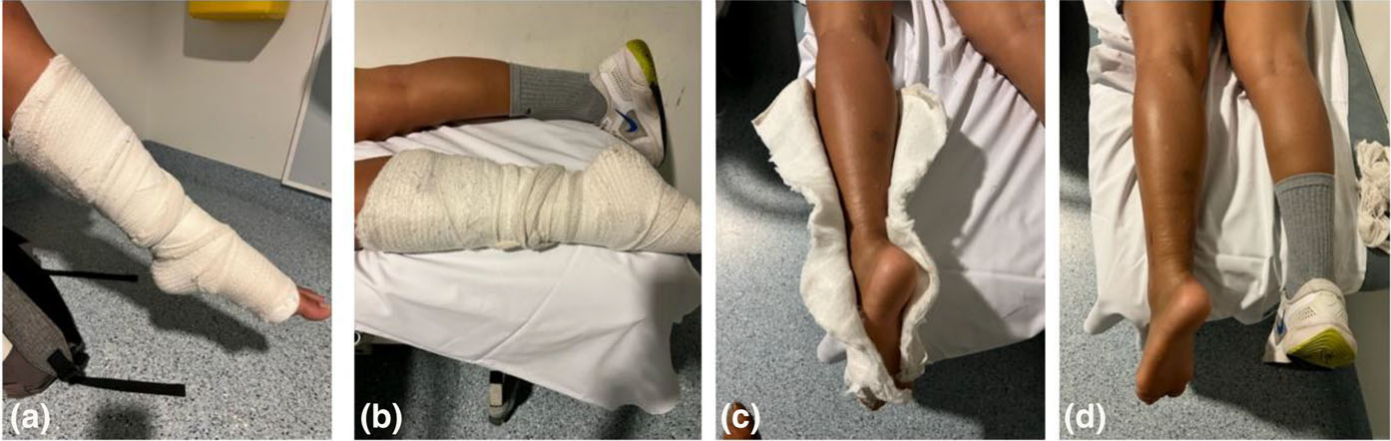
Immobilisation for a clinically suspicious ruptured Achilles tendon. (a) A front slab with the foot in plantar flexion. (b) For sonographic imaging, the patient is positioned prone with their foot hanging over the end of the bed. (c) The front slab is cut at the posterior aspect to allow visual inspection and sonographic imaging. (d) The front slab is removed to allow dorsiflexion and plantar flexion during sonographic imaging.
